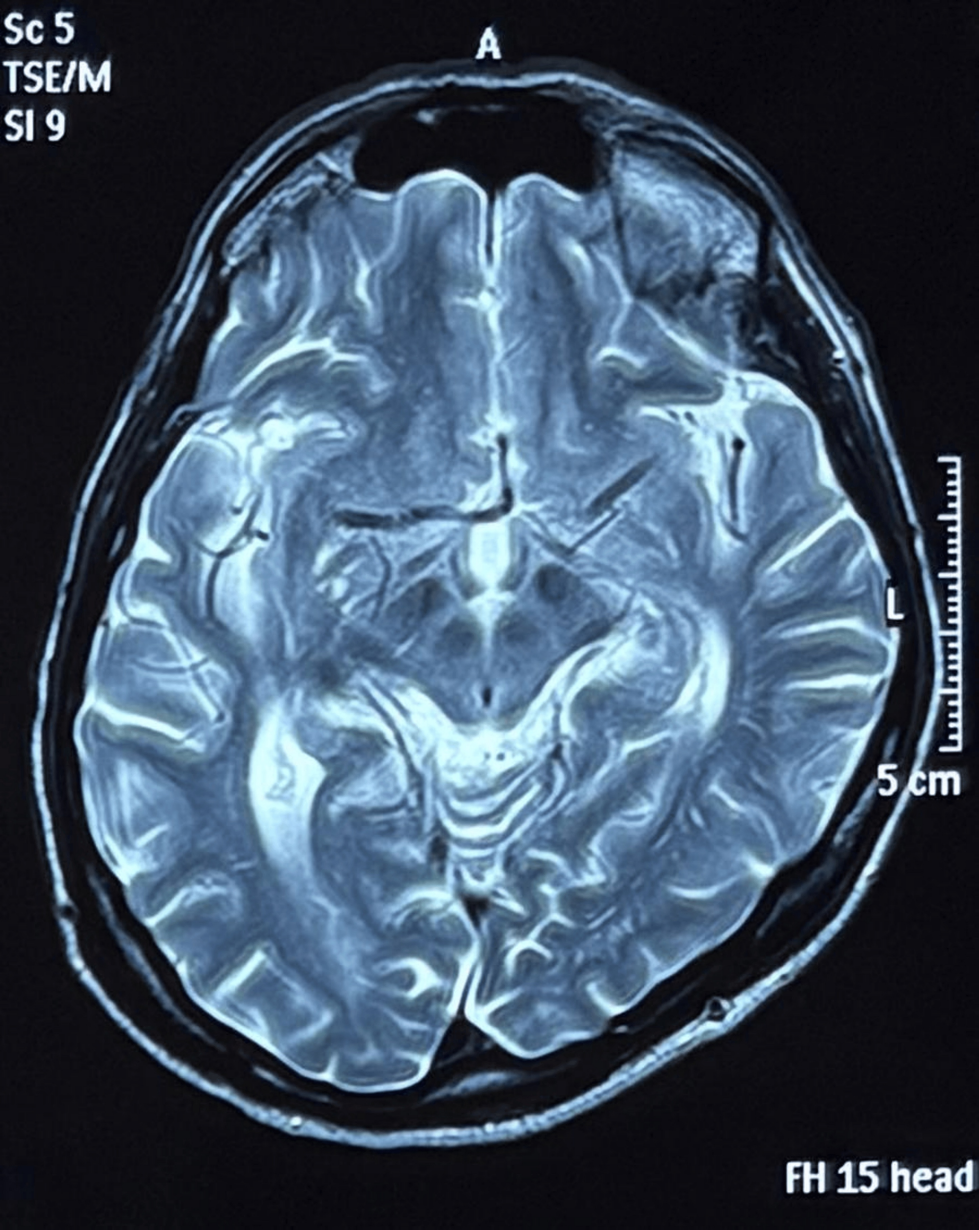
T2-weighted (T2W) magnetic resonance imaging (MRI) showed hyperintense lesion at the level of right pons
